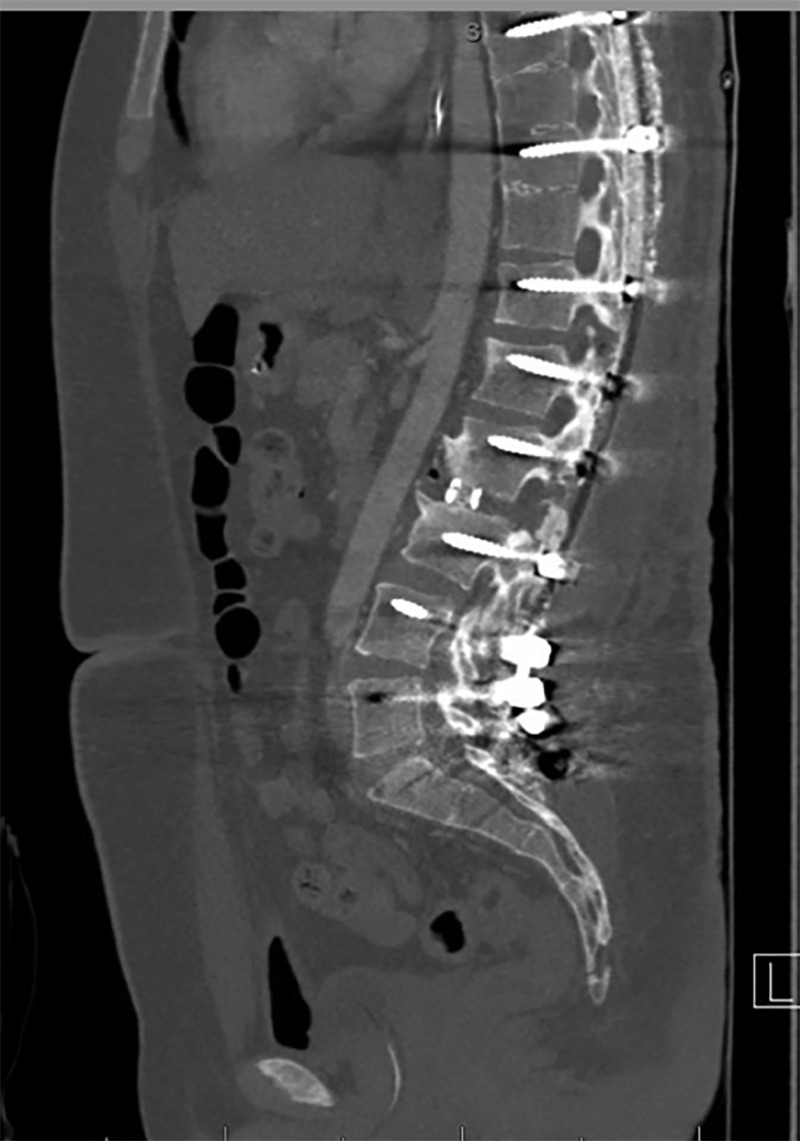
Postoperative Sagittal CT Image Immediate postoperative sagittal CT image demonstrating satisfactory placement of hardware, and complete correction of kyphotic deformity using Ponte osteotomy and posterior interbody fusion technique
